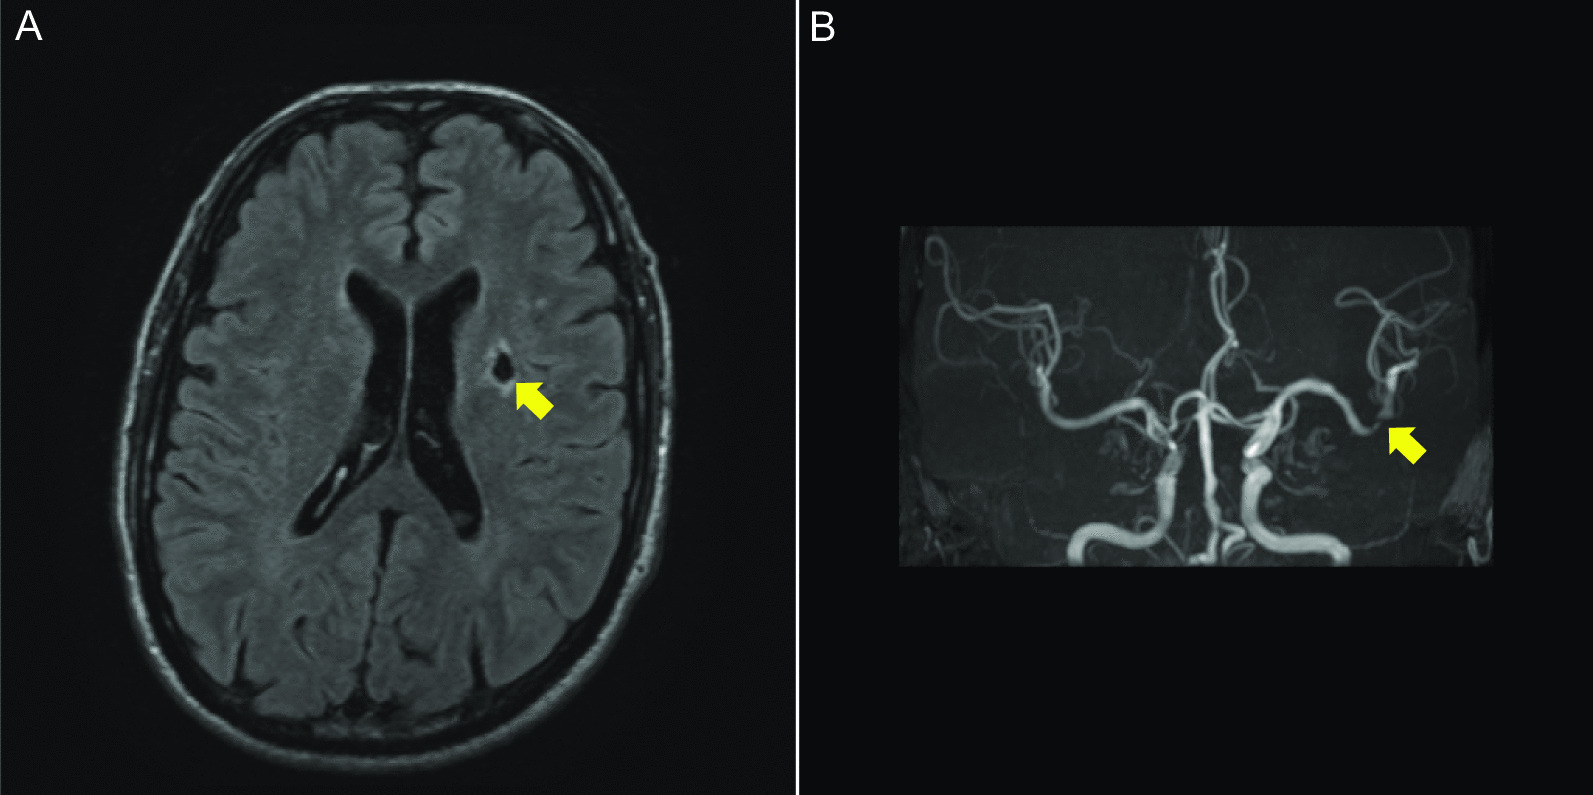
Magnetic resonance images of the brain 3 months prior to myocardial infarction. A Axial T2-weighted FLAIR MRI showing low intensity of the corona radiata (arrow). B Frontal view from magnetic resonance angiography showing stenosis of the left middle cerebral artery (arrow)
